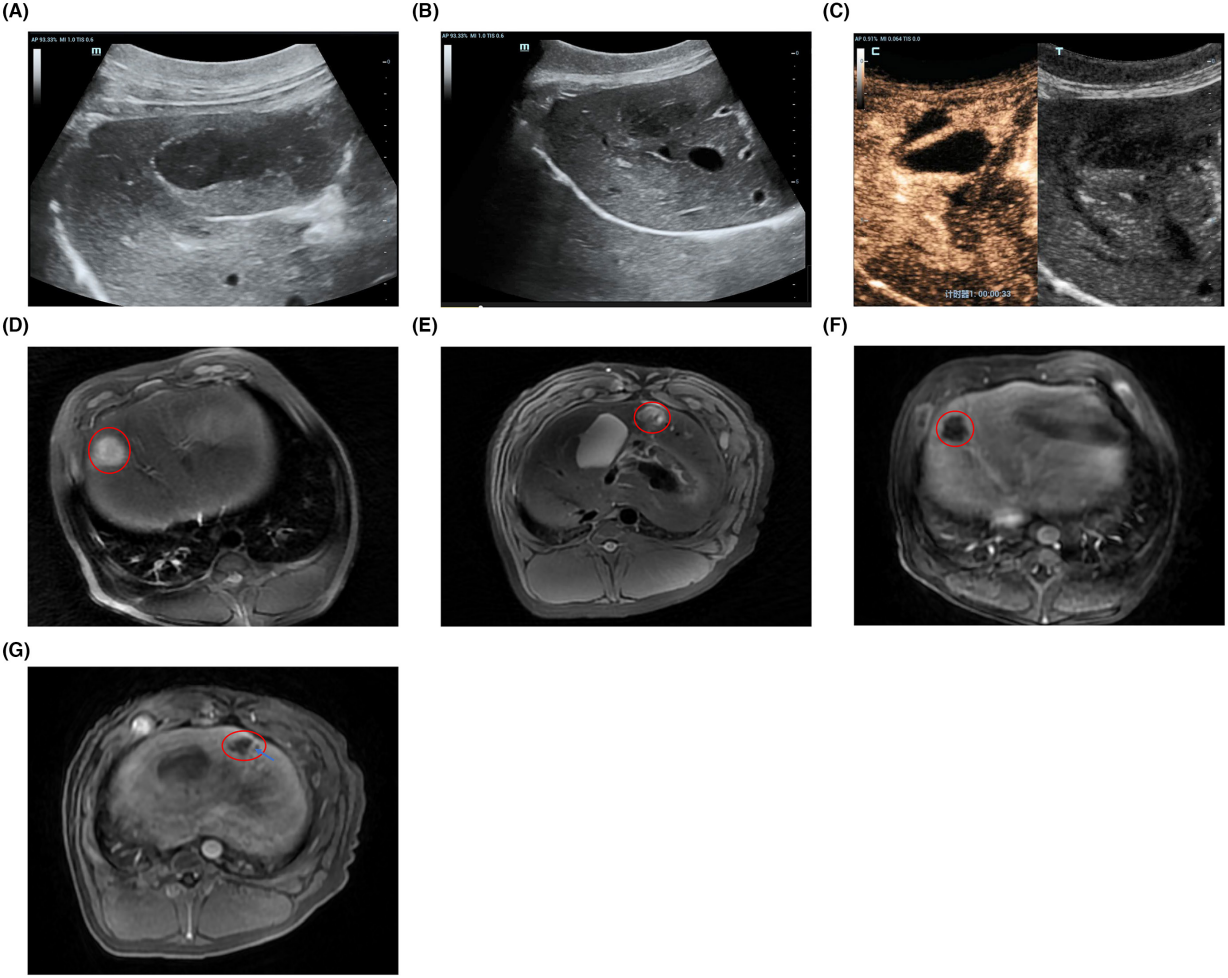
(A) Ultrasound was performed 2 days after H‐FIRE. On ultrasound images, the ablation area was hypoechoic, and the shape and margin were clearer. (B) The blood vessels passing through the ablation region were intact. (C) Two days after H‐FIRE, CEUS examination showed that the ablation region was completely non‐enhanced; however, the major blood vessels passing through this region still showed a normal enhancement pattern. (D) Contrast‐enhanced MRI was performed 2 days after H‐FIRE. The ablation areas appeared to be elliptical regions with high signal intensity on T2WI, and the boundary was clear. (E) The major blood vessel passing through the ablation area was intact on T2WI. (F) The ablation region showed no enhancement during all three phases. (G) The enhancement patterns of the major blood vessels passing through the ablation area were normal. (The ablation areas were indicated by red circles).
